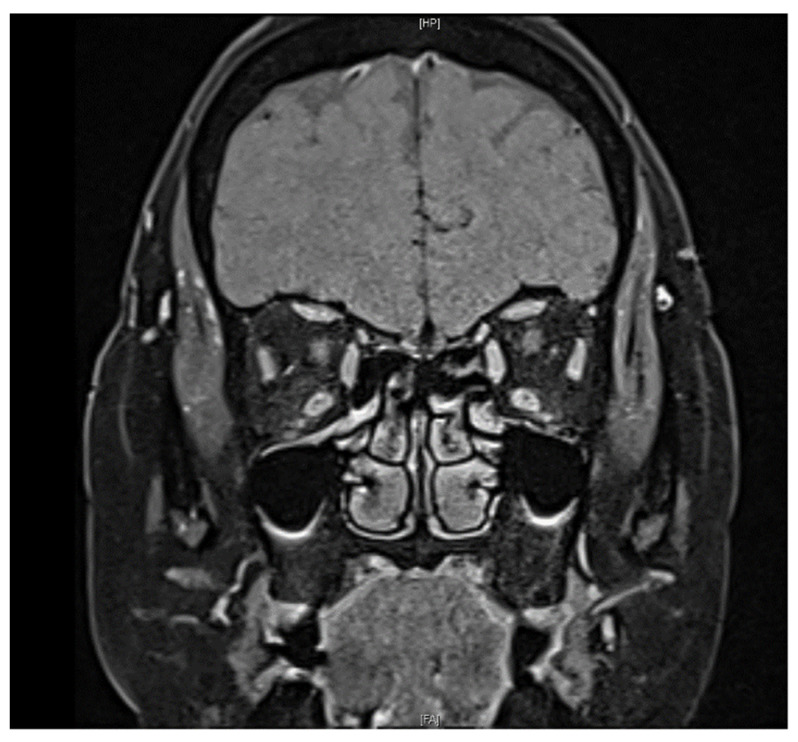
Fat-suppressed T1 coronal image on MRI revealed no contrast enhancement of the optic nerve in both eyes.
